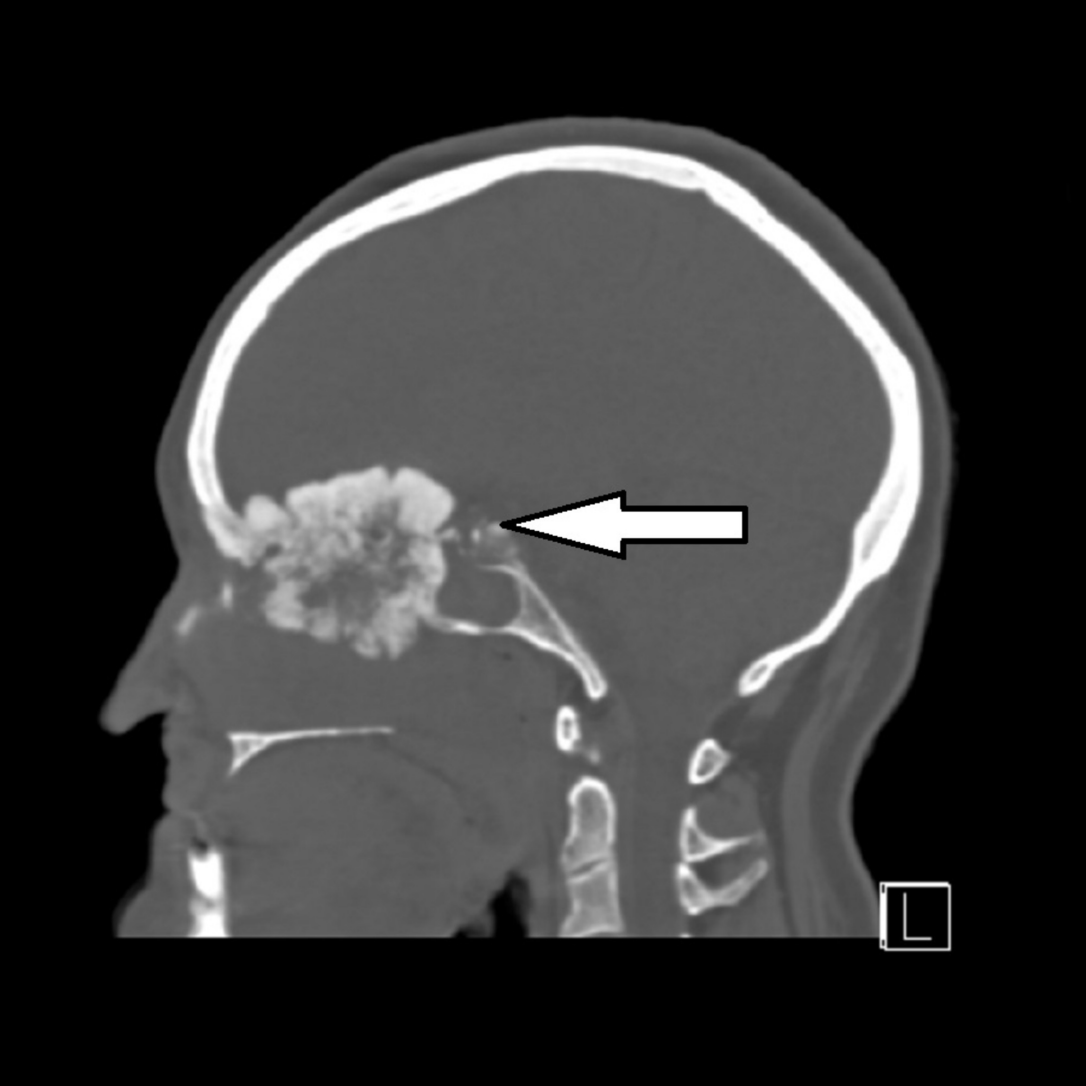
Sagittal non-contrast CT
